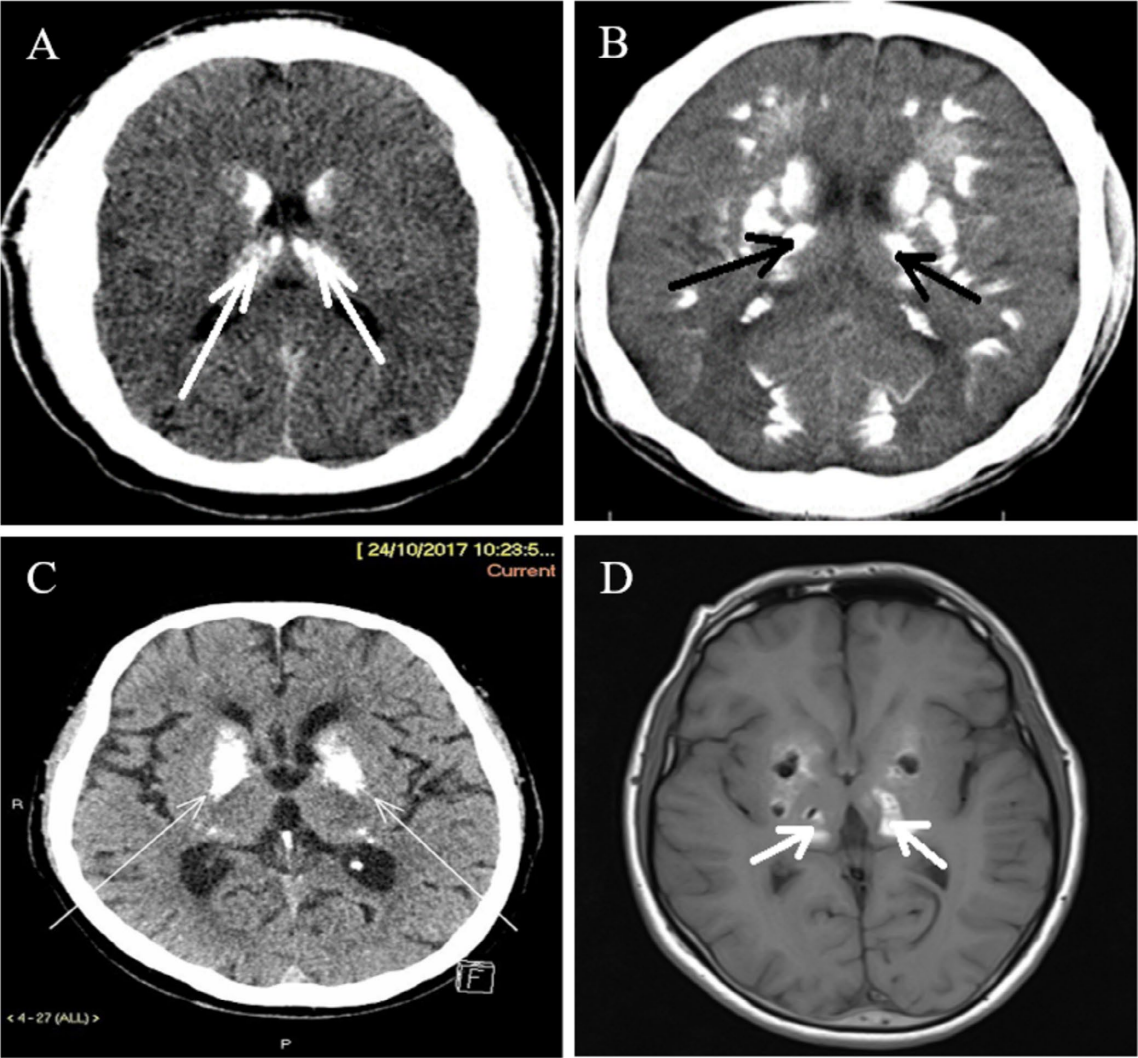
(A, B, C) CT scan of Fahr's disease patient shows bilateral symmetrical calcifications in the basal ganglia and subcortical white matter, characteristic of the disorder. (D) MRI of Fahr's disease patient, highlighting prominent calcium deposits in the basal ganglia, particularly in the globus pallidus and putamen region.
